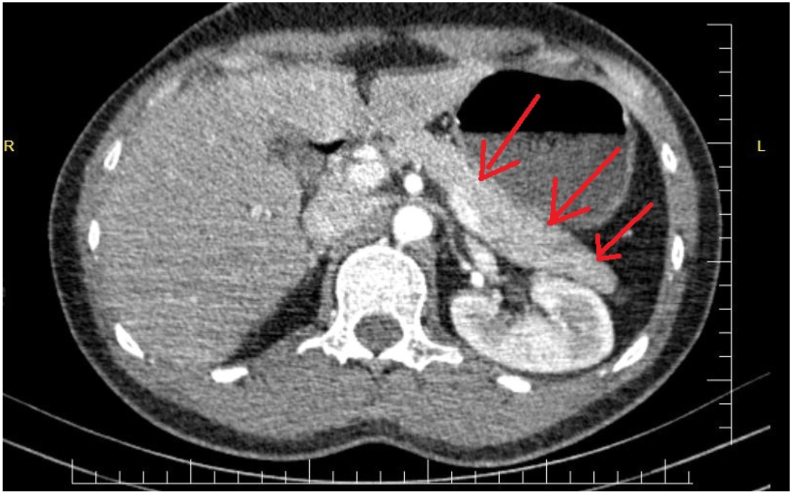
Axial abdominal CT scan with IV contrast at pancreatic level shows normal pancreas (arrows).
